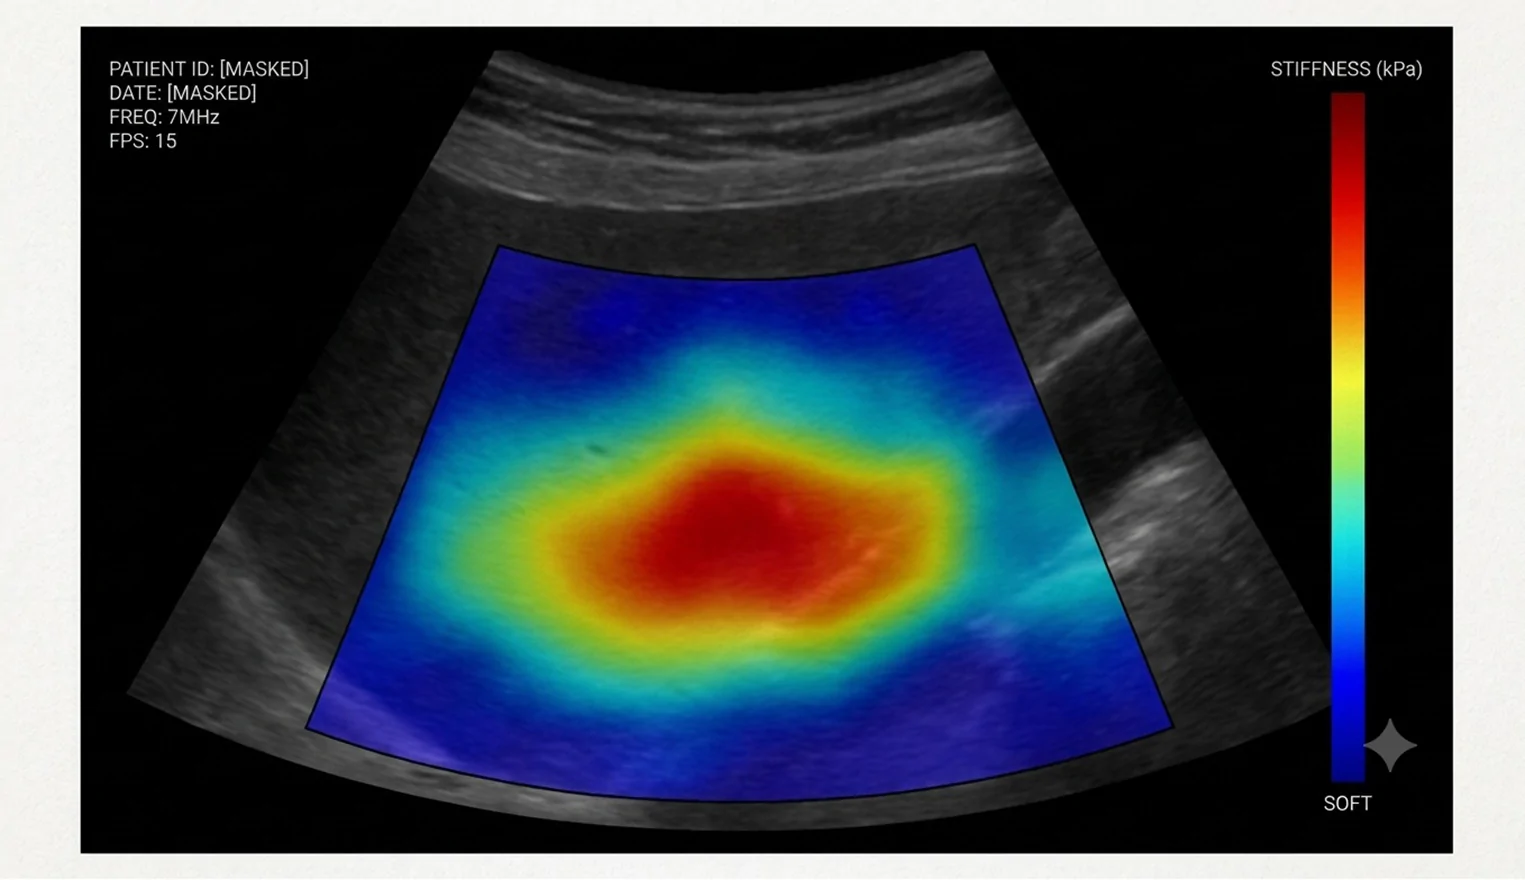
Grad-CAM overlay on liver ultrasound images for XAI based MASLD diagnosis.
